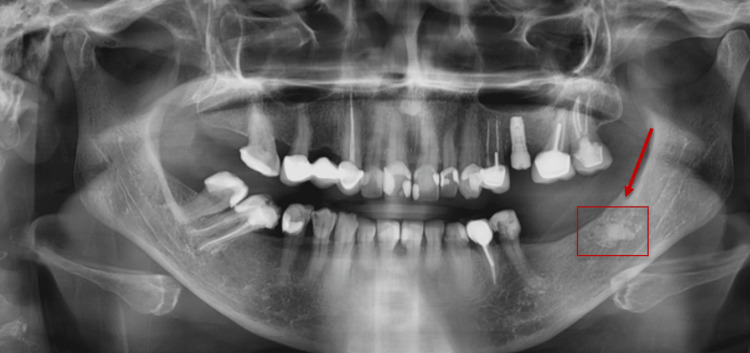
A panoramic radiograph of a 45-year-old female patient showing a radiopaque lesion on the left side of the mandibular edentulous posterior region.
